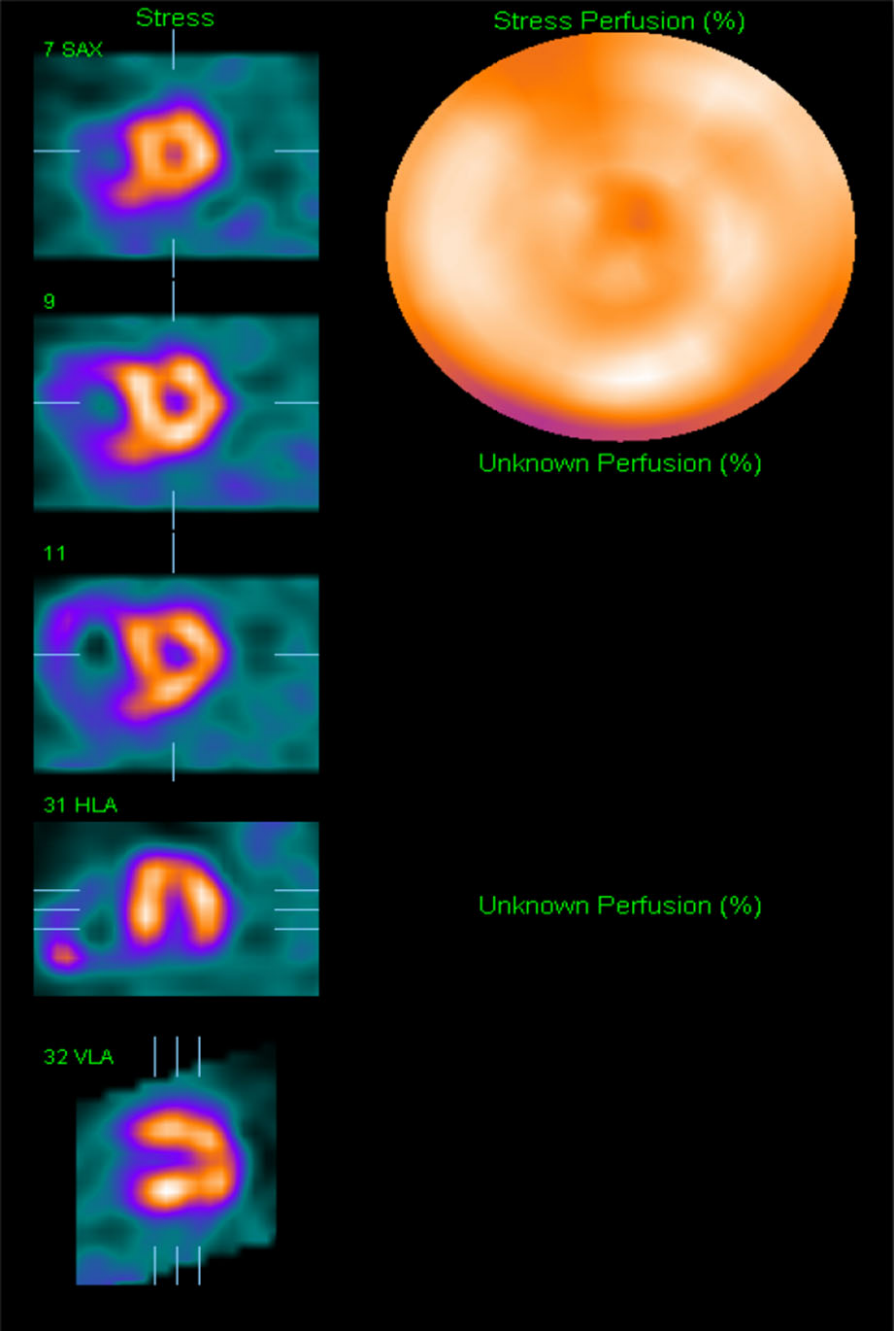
Case 4: Tc-99m tetrofosmin MPS of a 60-year-old woman with severe COPD and pulmonary fibrosis showing homogeneous stress perfusion throughout the left ventricular myocardium and signs of pulmonary hypertension. Resting images were not acquired. COPD, chronic obstructive pulmonary disease; MPS, myocardial perfusion scintigraphy; Tc, technetium
